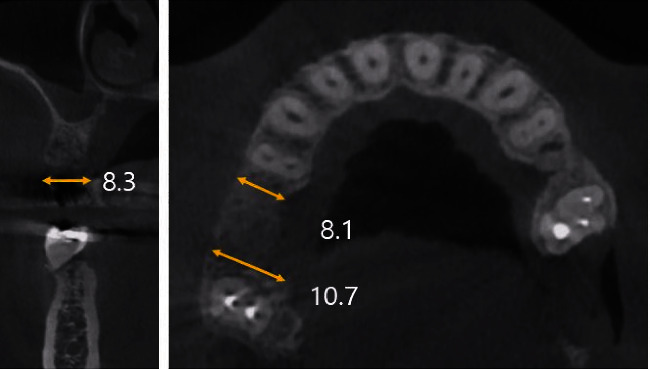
Postoperative CBCT view, showing that the width of the alveolar bone had increased (Case 3).
